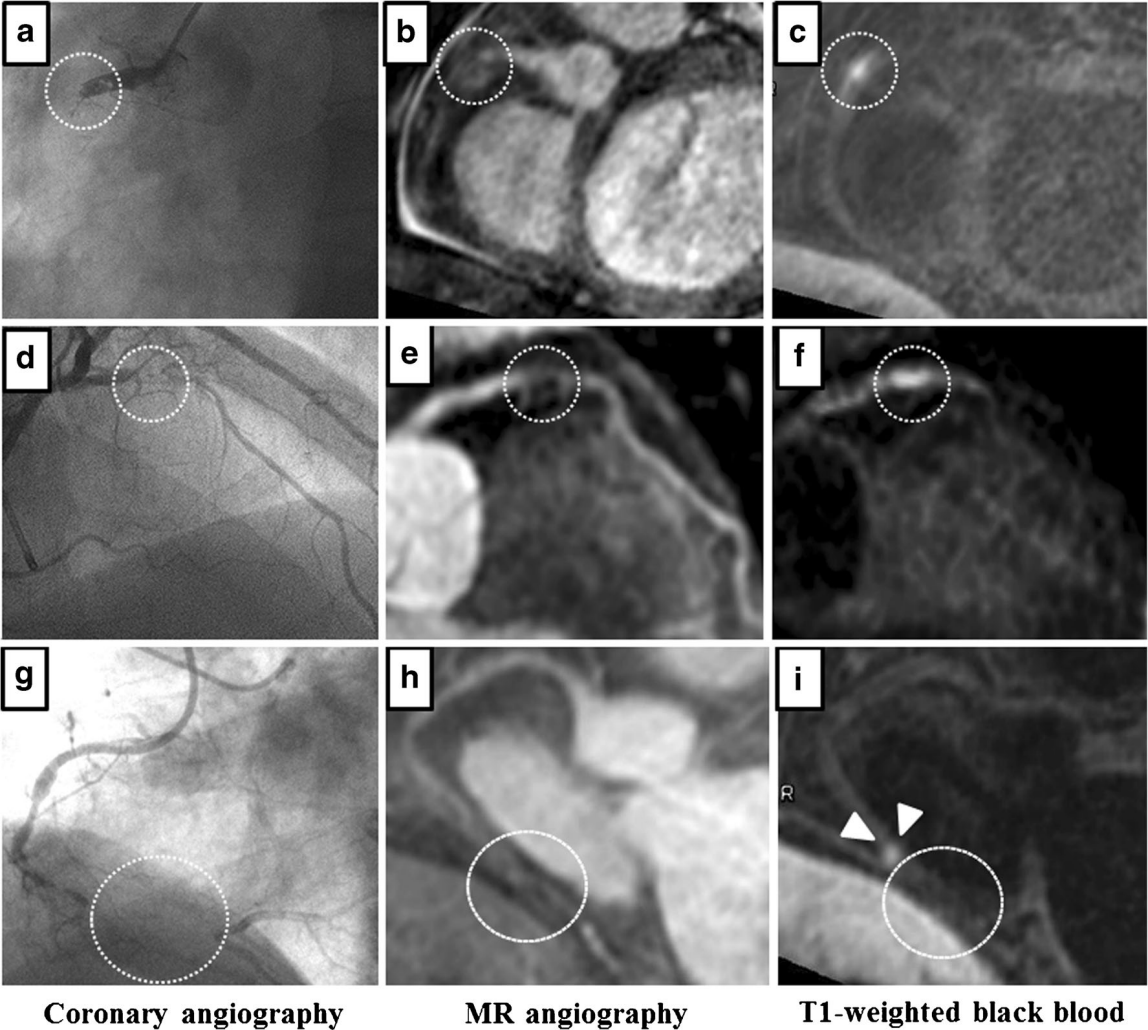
Representative angiographic images from patients with total coronary occlusion. a–c Coronary subacute total occlusion in a patient with an occlusion estimated duration of 14 days. a Total occlusion of the proximal right coronary artery (RCA) demonstrated on CAG (*dotted circle*). b Whole-heart MR coronary angiography showing absence of a blood signal at the same site (*dotted circle*) and a poor blood signal at the distal RCA from a collateral artery. c A HIS is shown at the occlusion site in a T1-weighted black-blood image (*dotted circle*). d–f SD-CTO in a patient with an occlusion estimated duration of 90–120 days. d Total occlusion of the mid left anterior descending artery (LAD) demonstrated on CAG (*dotted circle*). e Whole-heart MR coronary angiography revealed a lack of blood signal at the same site (*dotted circle*), whereas a good blood signal from a collateral artery was detected at the distal LAD site. f T1-weighted black-blood image demonstrated HIS at the region in interest (*dotted circle*). g–i LD-CTO in a patient with an occlusion estimated duration of more than 2 years. g CAG revealed a total occlusion at the distal RCA site (*dotted circle*). h The blood signal was absent in the same lesion on whole-heart coronary MR angiography (*dotted circle*), whereas the posterior descending artery was visible because of the presence of a collateral artery. i Absence and presence of a HIS at the occlusion (*dotted circle*) and proximal sites (*arrowheads*), respectively
